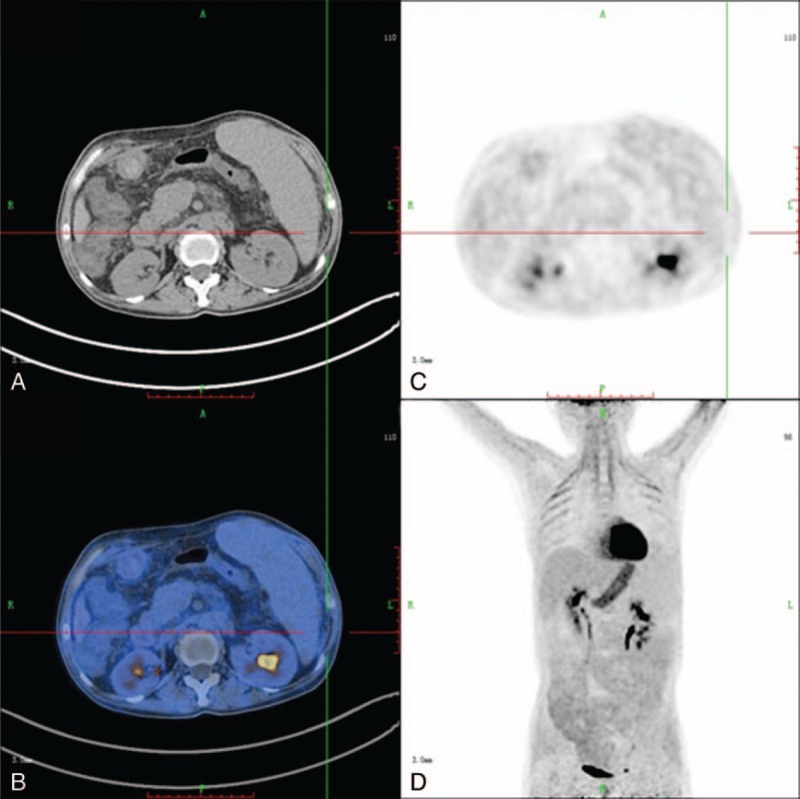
Images of 42-year-old woman who presented with fever for 1 week: axial CT (A), axial PET (B), axial fused PET/CT (C), and 3D PET (D). The patient had history of hepatic sclerosis. PET/CT images show peritoneal thickening and ascites. PET/CT images show normal uptake in the peritoneum. CT = computed tomography, PET = positron emission tomography.
